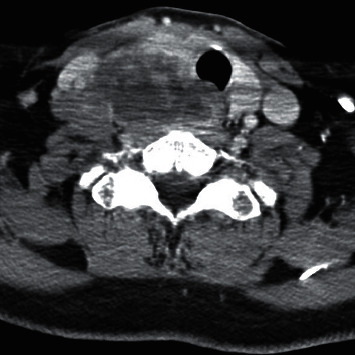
CT scan of the neck at presentation (axial image).
